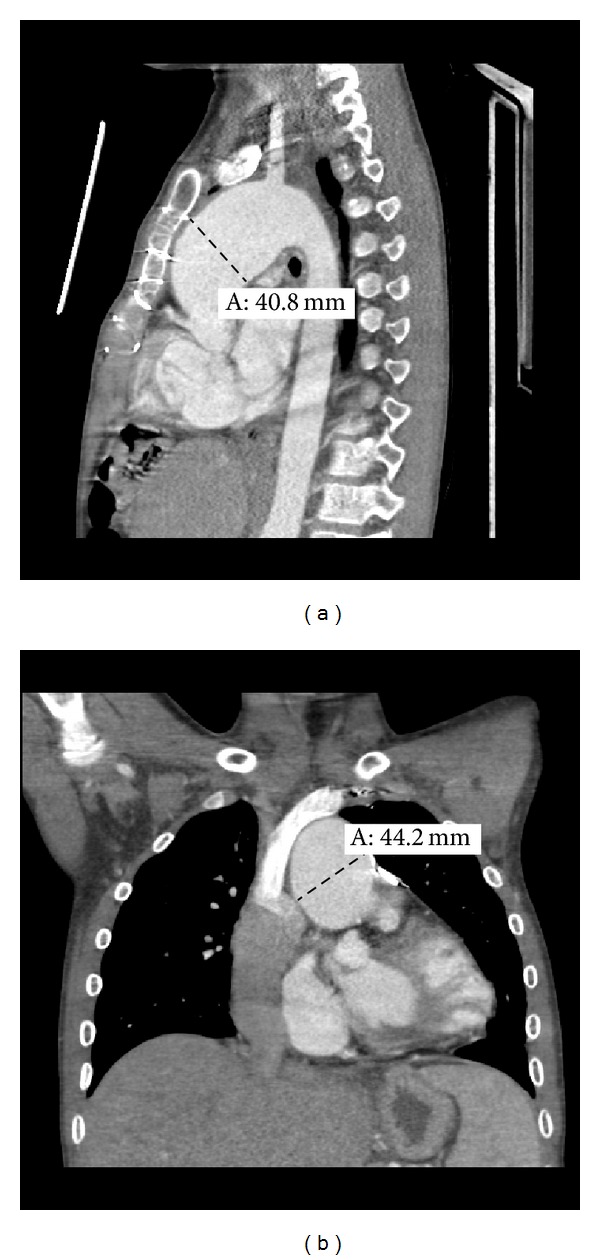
Optiray contrast-enhanced computed tomography images of the chest with sagittal (a) and coronal (b) reconstructions in the 14-year-old proband revealing aneurysmal dilation of the ascending aorta.
